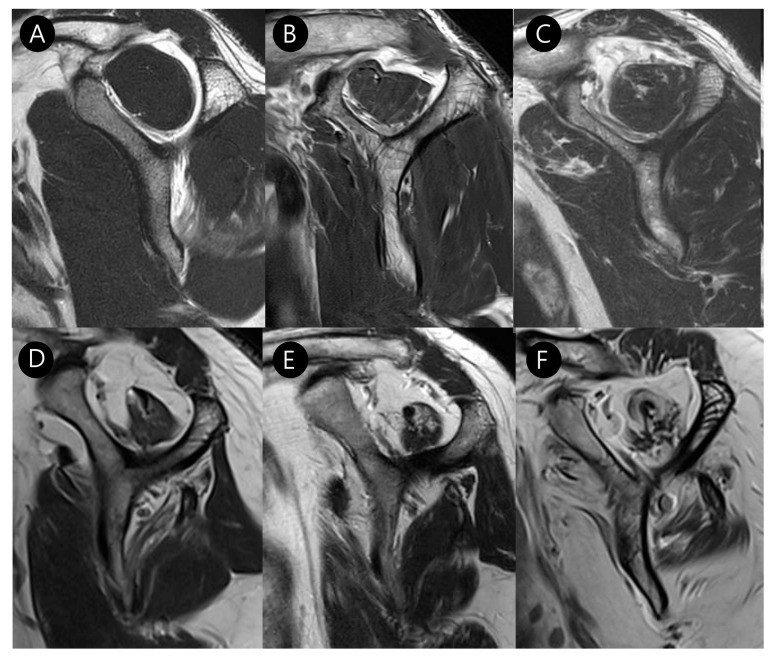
Evaluation of subscapularis muscle fatty infiltration on T2-weighted oblique sagittal images according to the modified Goutallier method [14]: (A) Grade 0: no fat. (B) Grade 1: some fatty streaks. (C) Grade 2: less fat than muscle in the upper half of the muscle. (D) Grade 3: more fat than muscle in the upper half of the muscle, with normal or fatty streaks in the lower half of the muscle. (E) Grade 4: more fat than muscle in the upper half of the muscle, with less fat than muscle in the lower half of the muscle. (F) Grade 5: more fat than muscle in the upper and lower halves of the muscle.
